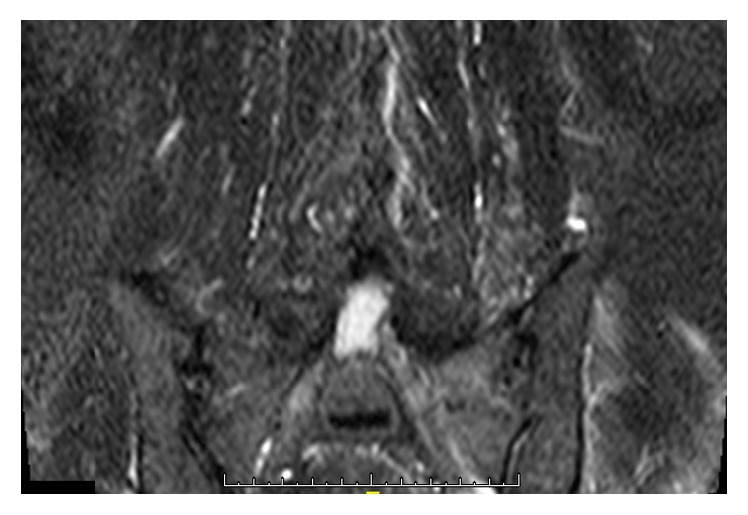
MRI with osteomyelitis of the sacrum as well as free air bubbles in the sacrum.
